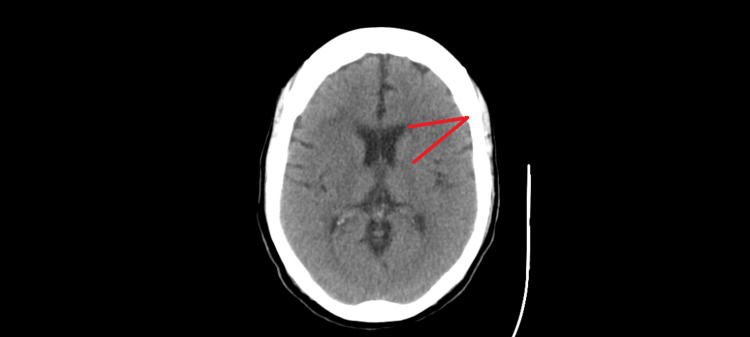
CT head without contrast Two merging lines indicate areas of possible demyelination on an axial view of the brain.
